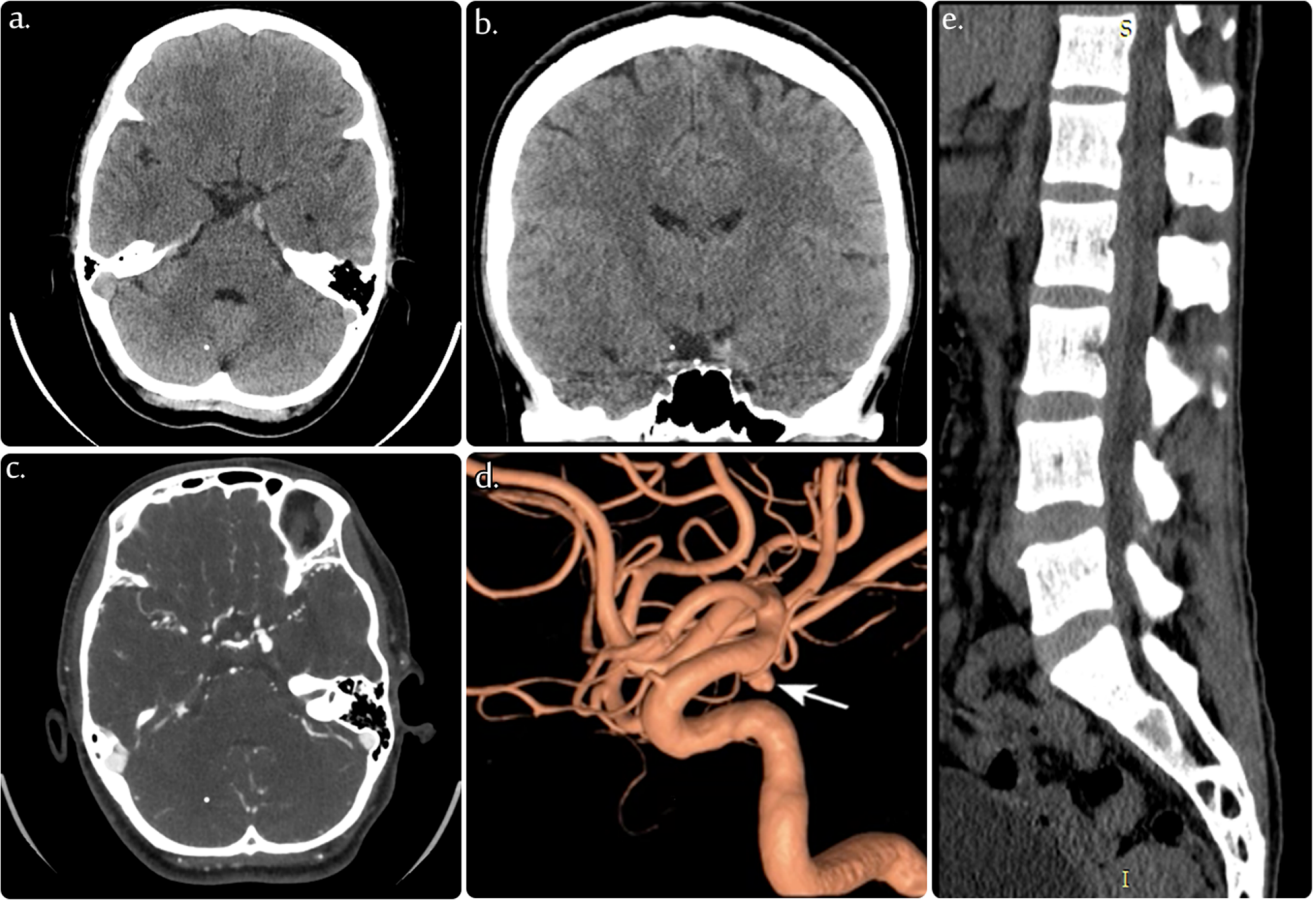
a, b, c Selective images of axial and coronal unenhanced computed tomography (CT) of the brain showing a small subdural hematoma at the anterior edge of the left tentorium, medial to the left uncus. d Selective 3D image of the left internal carotid artery (ICA) demonstrating unilocular posterior communicating artery (PCOM) aneurysm. (e) Selective image of the sagittal CT of the lumbar spine showing a subdural hematoma exerting a mass effect on the spinal canal
